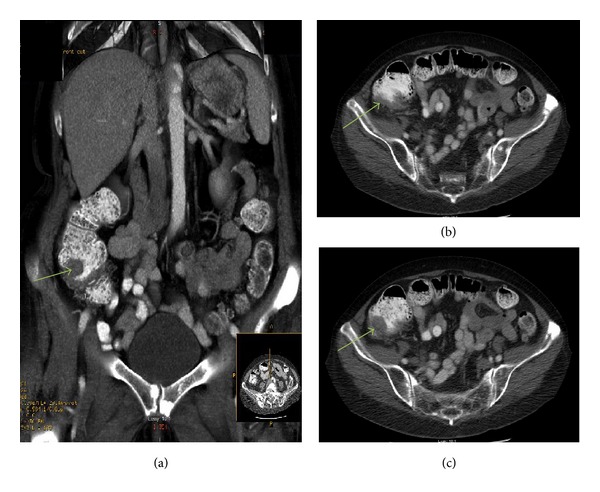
CT abdomen and pelvis. (a) (coronal view) and (b) and (c) (axial views) show an area of irregular wall thickening in the cecum (green arrows).
